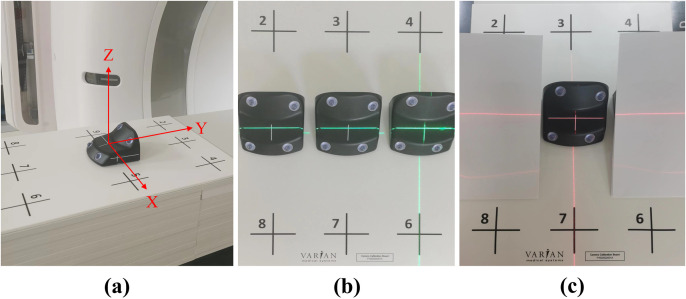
The proposed calibration method. (a) CT coordinate system; (b) Initial setup; (c) Calibration for position 1.
